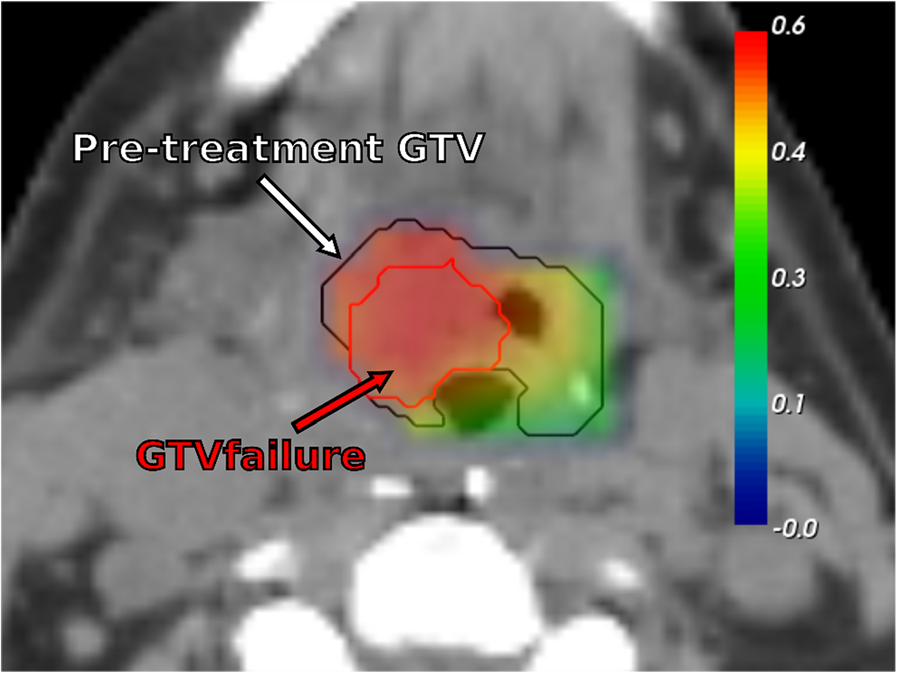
Example of probability map of recurrence, generated with random forest, overlaid on the pre-treatment CT. Black contour: pre-treatment GTV. Red contour: GTVfailure
